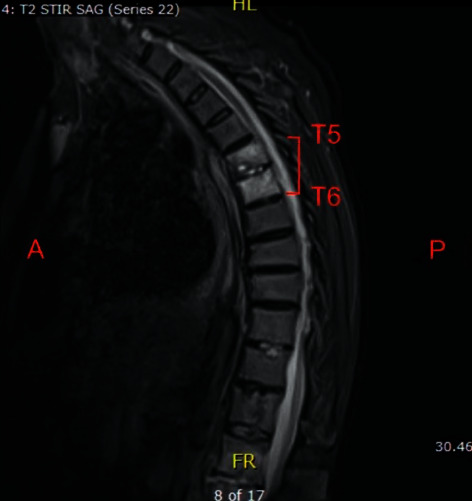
MRI thoracic spine with findings of discitis/osteomyelitis involving T5-6 with trace enhancement of the left anterior T5-6 epidural space.
